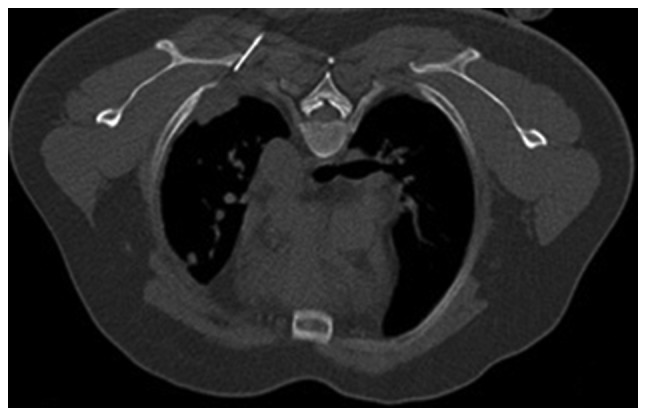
Male, aged 39 years, the first result of the computed tomography-guided cutting needle pleural biopsy was a false-negative lesion; the secondary biopsy led to a diagnosis of metastatic adenosquamous carcinoma.
